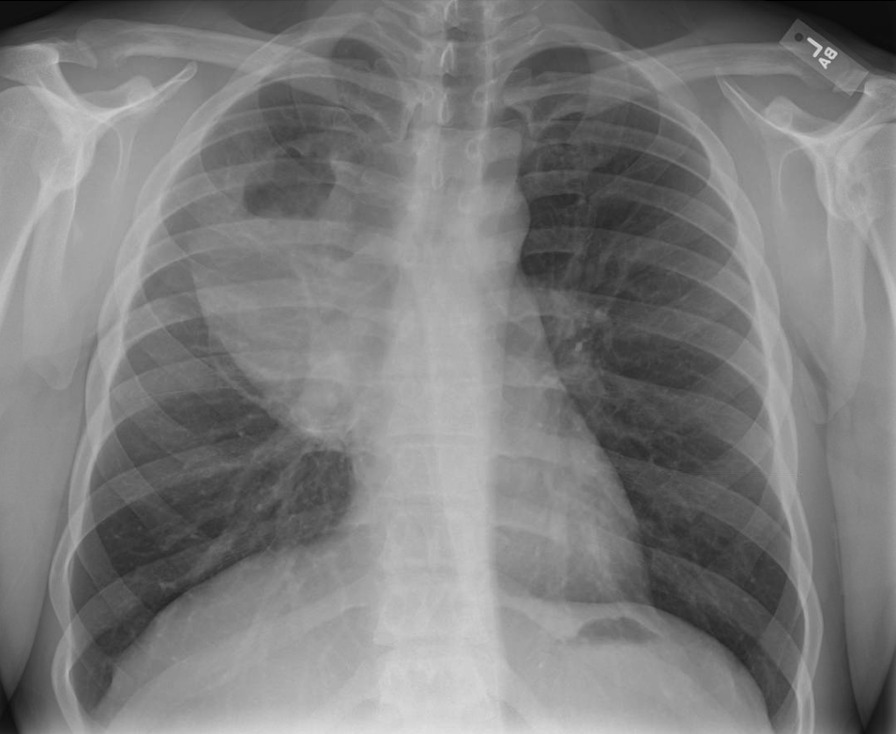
Initial chest X-ray (PA view). Right upper lobe cavitary lesion
